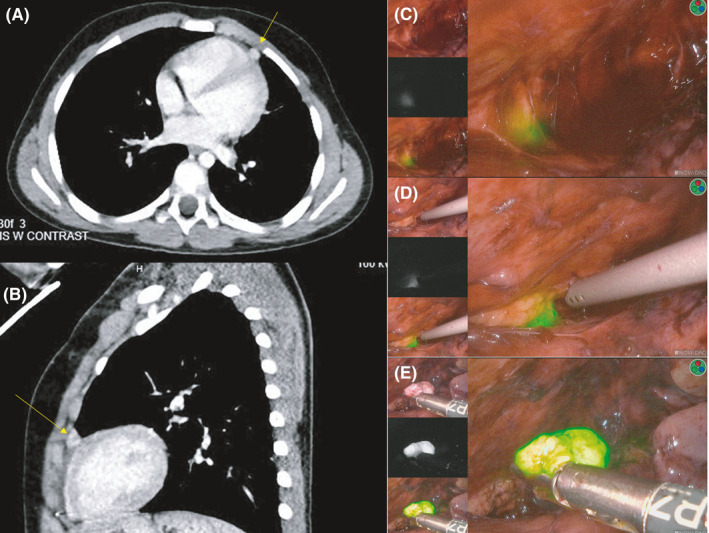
CT and intraoperative imaging of HB pericardial lesion. The left panel (A‐B) demonstrates the preoperative CT in axial (A) and sagittal (B) views. The lesion is anterior to the heart, indicated by the yellow arrow. The right (C‐E) panel shows the intraoperative imaging during thoracoscopy. Each of the intraoperative images shows the three views available in the operating room with the Pinpoint ICG system (top – normal camera view, middle – black and white with ICG avidity appearing white, bottom/right – ICG overlay view where ICG‐avidity appears green). The top image (C) shows the initial visualization of the pericardial lesions and the following images (d&e) demonstrate the increasing ICG avidity with progressive dissection and mobilization. This highlights the penetration depth of ICG and the visual enhancement accomplished with additional dissection
